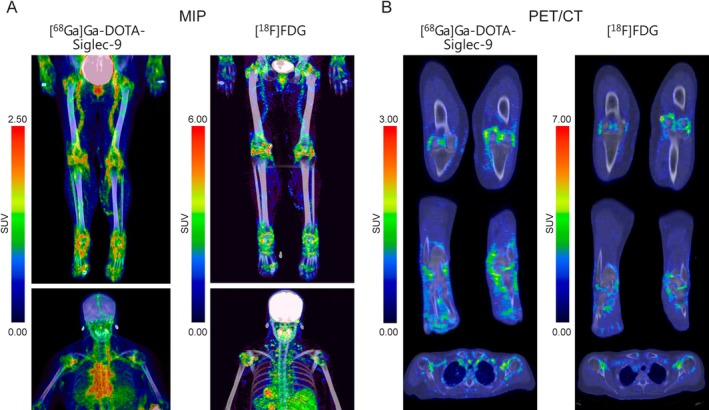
(A) Maximum intensity projection (MIP) images demonstrating increased bilateral tracer uptake in the knees, ankles, and metatarsophalangeal joints (upper panels) and shoulders (lower panels). (B) Representative fused positron emission tomography/computed tomography (PET/CT) cross‐sectional images of the knees (upper images), ankles and metacarpophalangeal joints (middle images), and shoulders (lower images), demonstrating increased [^68^Ga]Ga‐DOTA–Siglec‐9 uptake corresponding to clinically and ultrasonographically confirmed active synovitis. [^18^F]FDG, ^18^F‐labeled fluorodeoxyglucose; SUV, standardized uptake value.
